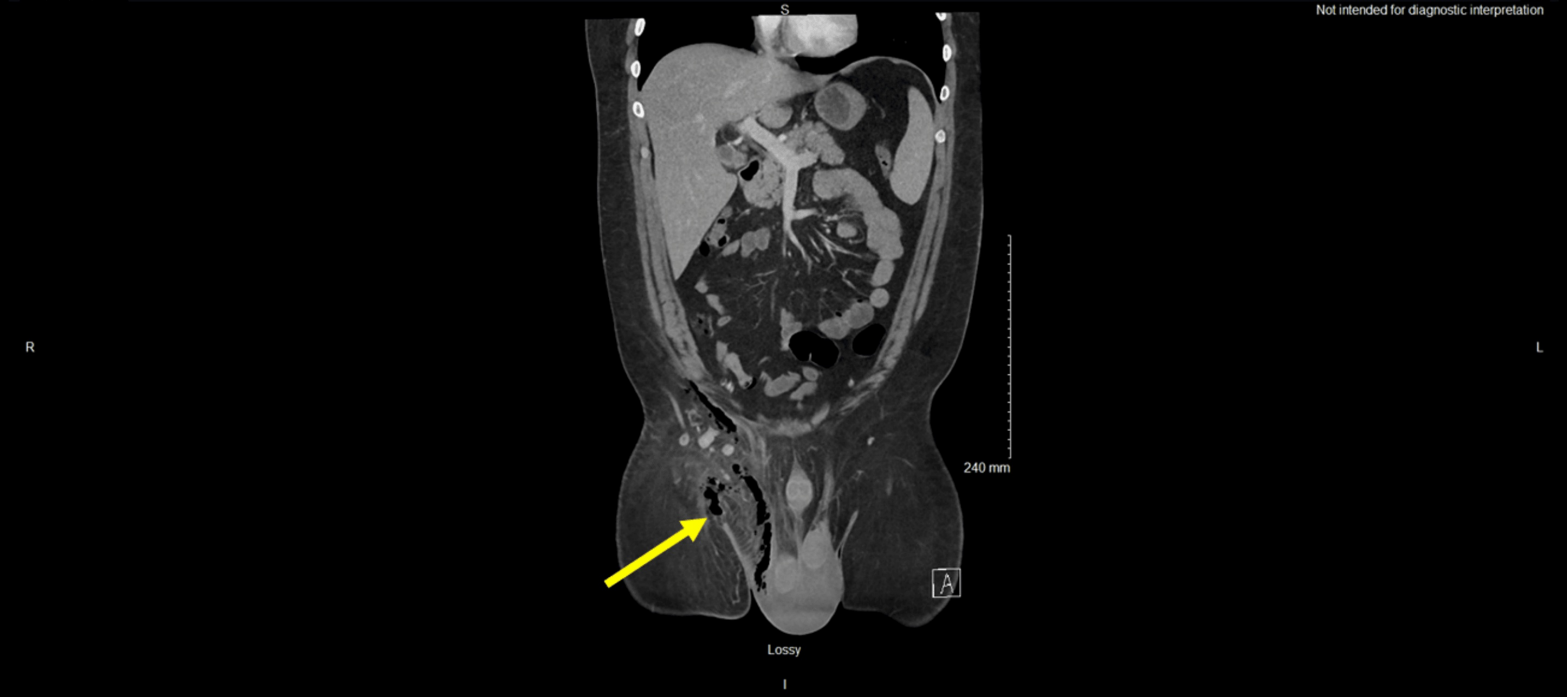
Coronal view of contrast-enhanced CT image demonstrating extension of subcutaneous gas from the right hemiscrotum into the inguinal region
